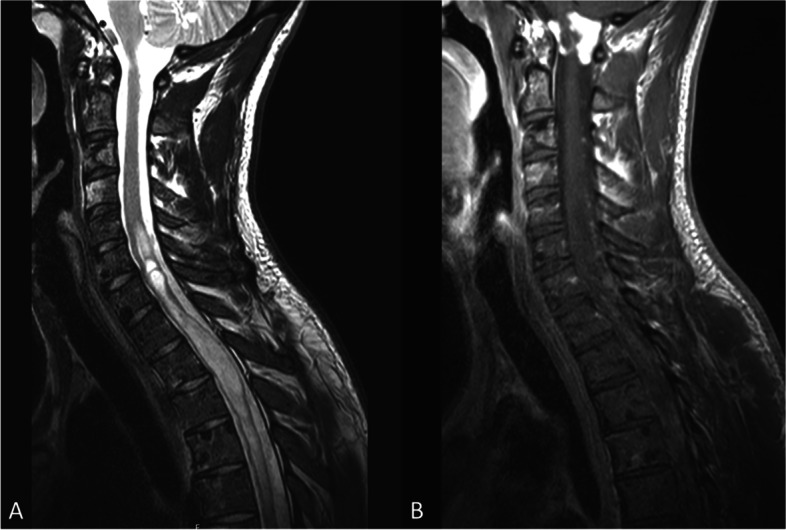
The initial MR spine image presents intra- and extra-medullary metastatic tumor lesions. A Sagittal T2-weighted MRI demonstrates diffuse long-distance bulky disease in the spinal cord, as well as diffuse intraosseous lesions. B Contrast-enhanced sagittal T1-weighted MRI of the spinal cord shows a contrast-enhanced metastatic lesion at the craniocervical junction
